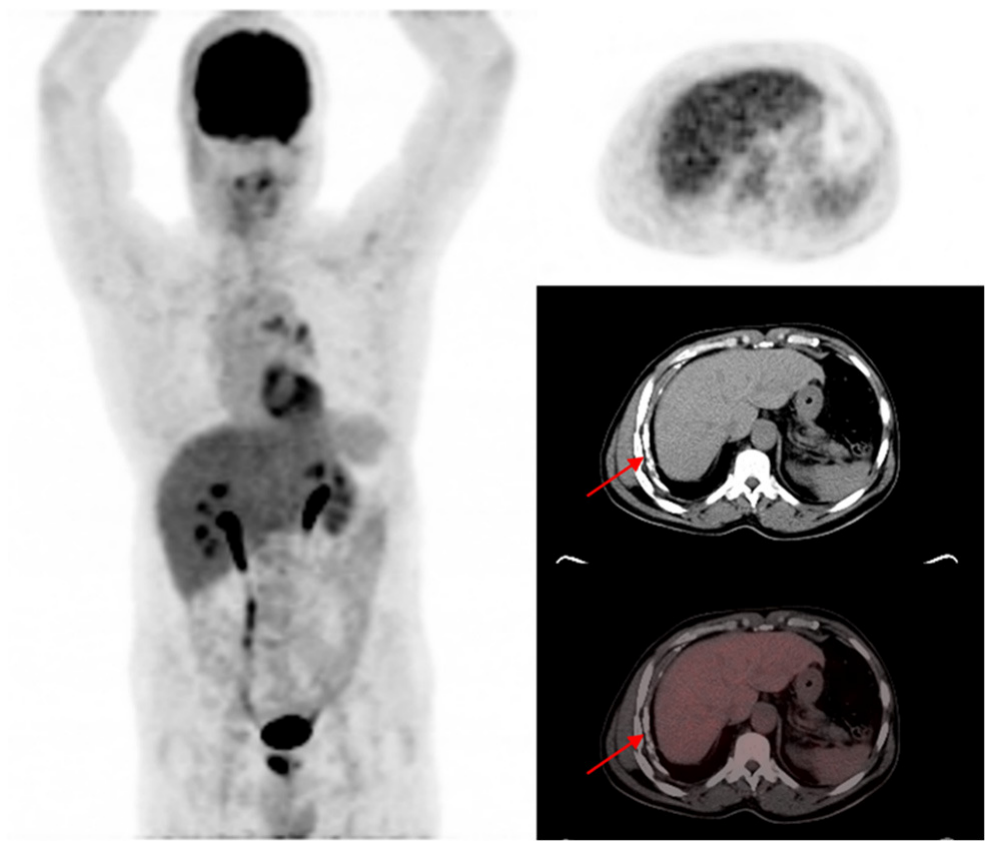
A 58-year-old HIV-infected male who completed a six-month course of anti-tuberculous medication for drug-sensitive pulmonary tuberculosis. The maximum intensity projection scan (MIPS) shown on the left does not demonstrate significant residual metabolic activity in the lung fields bilaterally. On the axial PET, CT, and fused PET/CT images shown on the right, pleural calcifications (red arrows) without corresponding significant residual metabolic activity is shown. Calcifications developed in this patient on the background of pleural effusion that complicated the active phase of the tuberculous disease.
